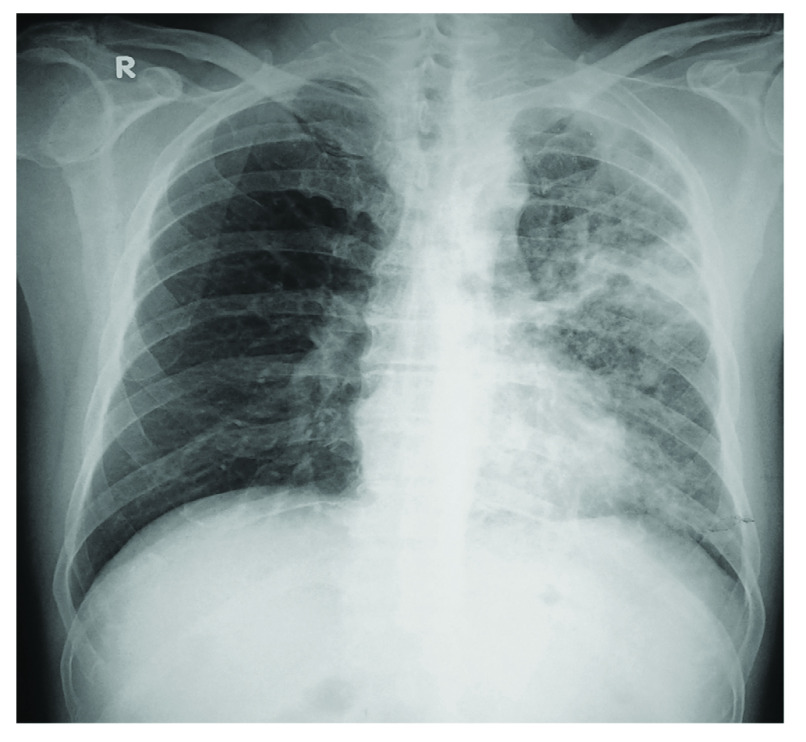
Initial chest X-ray of a 64-year-old man showing extensive changes in the left lung field, including small cavities
